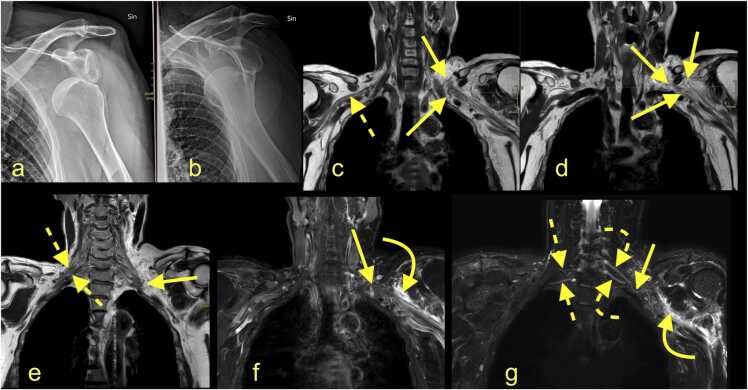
(a and b) A 43-year-old patient presented with an anterior shoulder dislocation on the left, which was quickly repositioned. Before repositioning, there was no function in the left elbow and hand. Magnetic resonance imaging of the left brachial plexus (BP) with (c-e) T2-weighted DIXON sequence, (f) T2-weighted DIXON, sequence with fat suppression, and (g) neurography showed total rupture of the left brachial plexus at the level of the cords. The stumps are significantly frayed (arrows), and the proximal stumps are thickened (curved dashed arrows). The area of rupture is surrounded by hematoma (curved arrows). The normal BP is on the right side (dashed arrows). Continued in Fig. 4.
